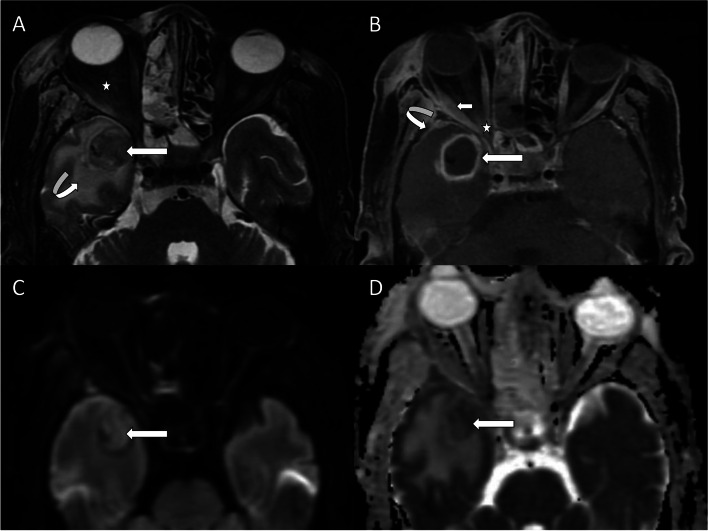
Orbital and cerebral involvement in ROCM. Axial fat-saturated T2-weighted image (A) shows a well-defined hypointense lesion (straight long arrow) in the right temporal lobe with surrounding edema (curved arrow). Right intraconal fat (white star) shows stranding with associated proptosis of the right eyeball. Axial contrast-enhanced fat-saturated T1-weighted image (B) shows peripheral enhancement of the temporal lobe lesion (straight long arrow) along with the enhancement of meninges (curved arrow). The right lateral rectus muscle appears bulky with heterogeneous enhancement (straight short arrow). Also note enhancing soft tissue in the right orbital apex (white star). The temporal lobe lesion (straight white arrows) in axial diffusion-weighted (C) and apparent diffusion coefficient (D) images shows central diffusion restriction, suggestive of an intracerebral abscess
